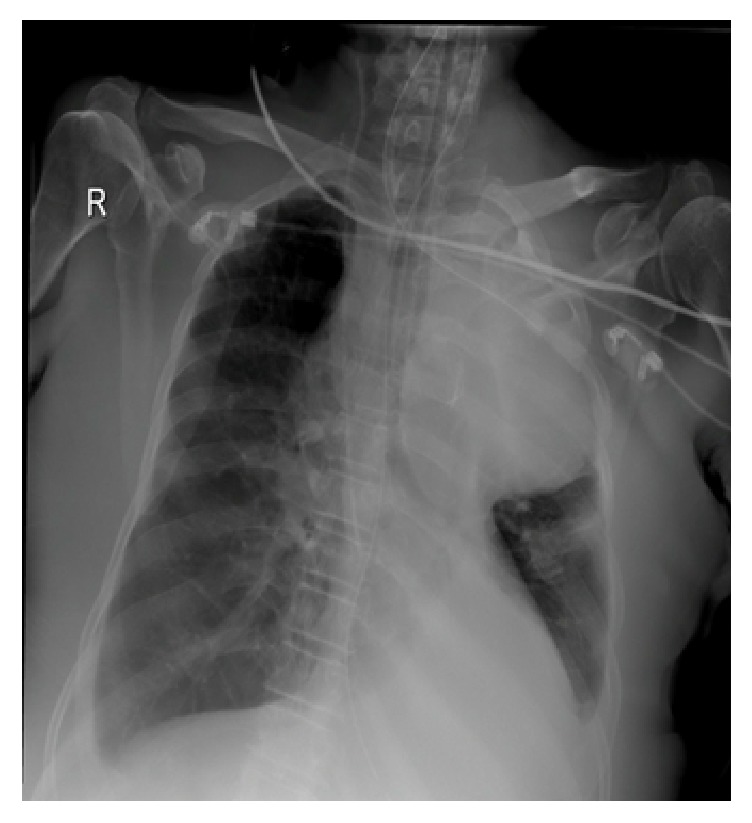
Chest X-ray shows left mediastinal mass.
